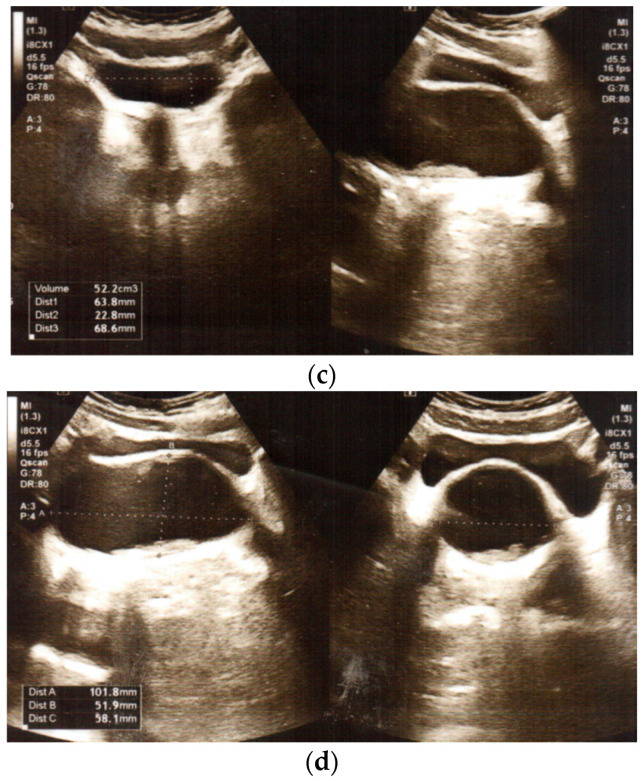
Transabdominal pelvic ultrasound at the time of diagnosis: (a,b) duplication of uterus: uterus with right-sided oval hypoechogenic structure, and normal ovaries (c,d) duplication of vagina, with haematocolpos on the right side.
